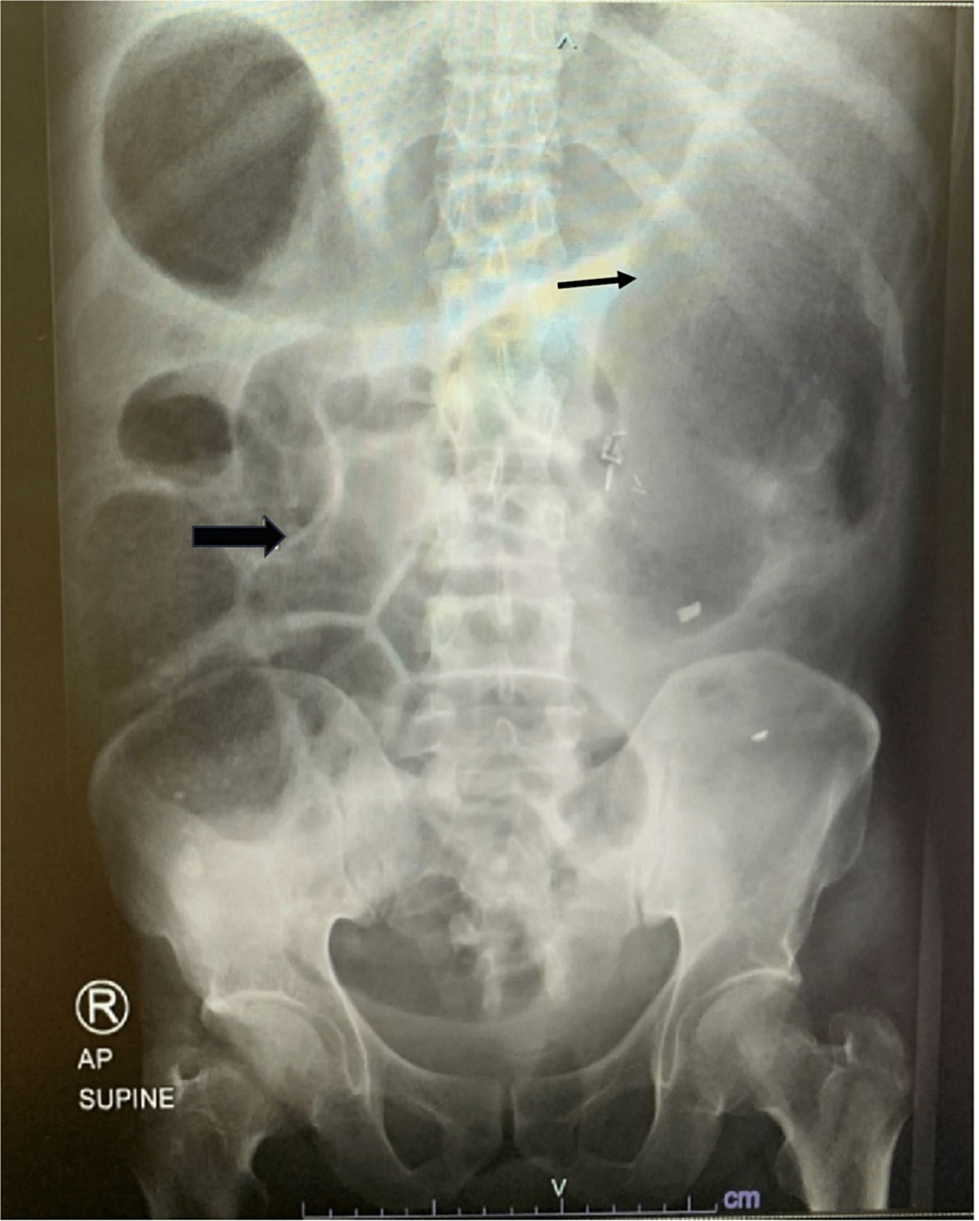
A follow-up plain abdominal (AP supine view) X-ray The image demonstrates a progression in distension of the large bowel loop with fecal matter (block arrow) with a probable transition point at the descending colon. The dilated descending colon measures approximately 13.5 cm in the maximum transverse diameter (line arrow).
